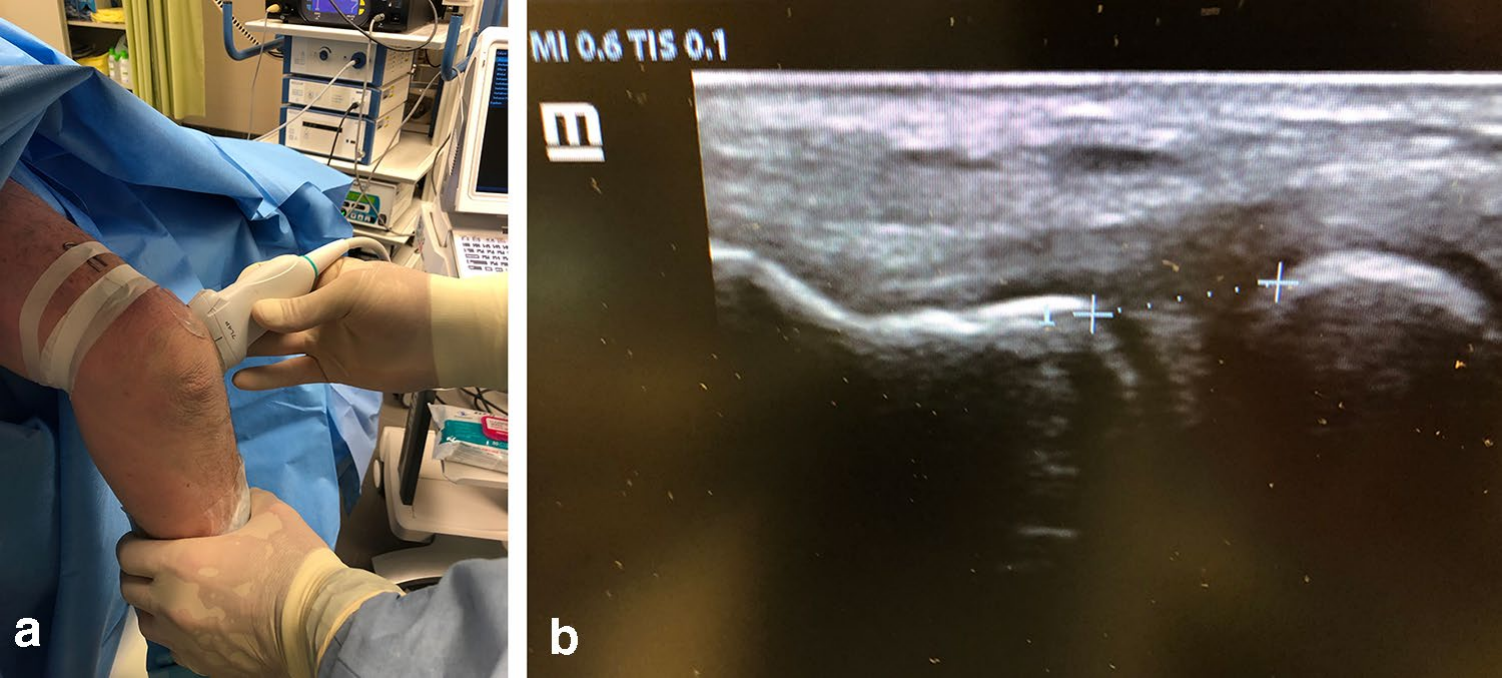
Ultrasound assessment of lateral stability. The probe was aligned to the lateral epicondyle and the extensor tendons (a). The shortest distance between the rim of the radial head and the capitellum was measured (b)
